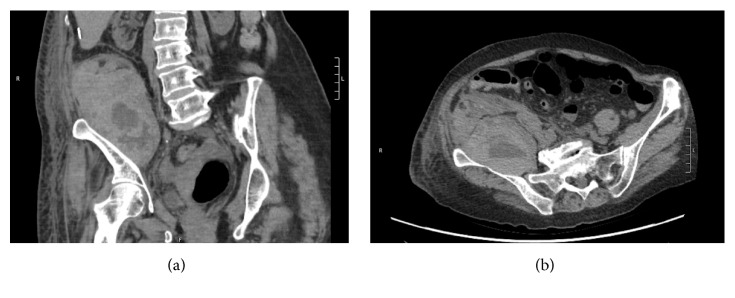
Computed tomography images without contrast in coronal plane (a) and axial plane (b) demonstrating right-sided iliopsoas hematoma as presented in case 1.
